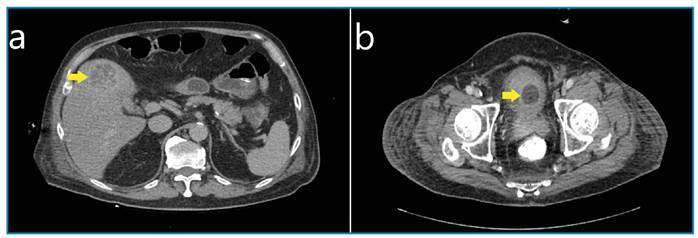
A CT scan of the abdomen and pelvis showing (a) a liver abscess in segment V measuring 3.9 x 3.6 x 2.9 cm and (b) a prostate abscess measuring 3.5 x 3.2 x 3.7 cm.
